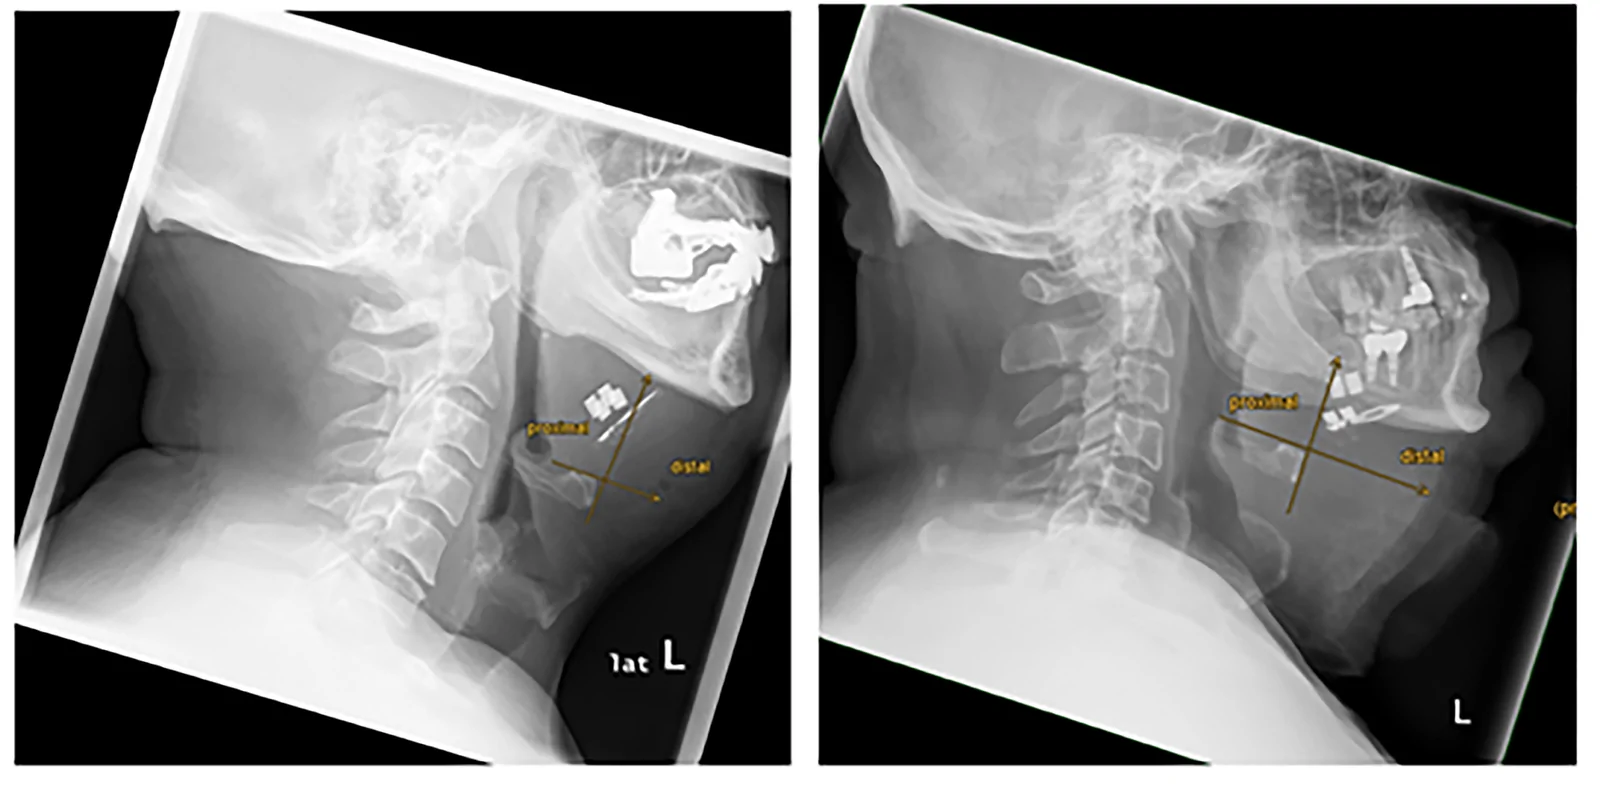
x-ray (lateral). Position of paddle electronics in relation to the anterior margin of the hyoid bone.
